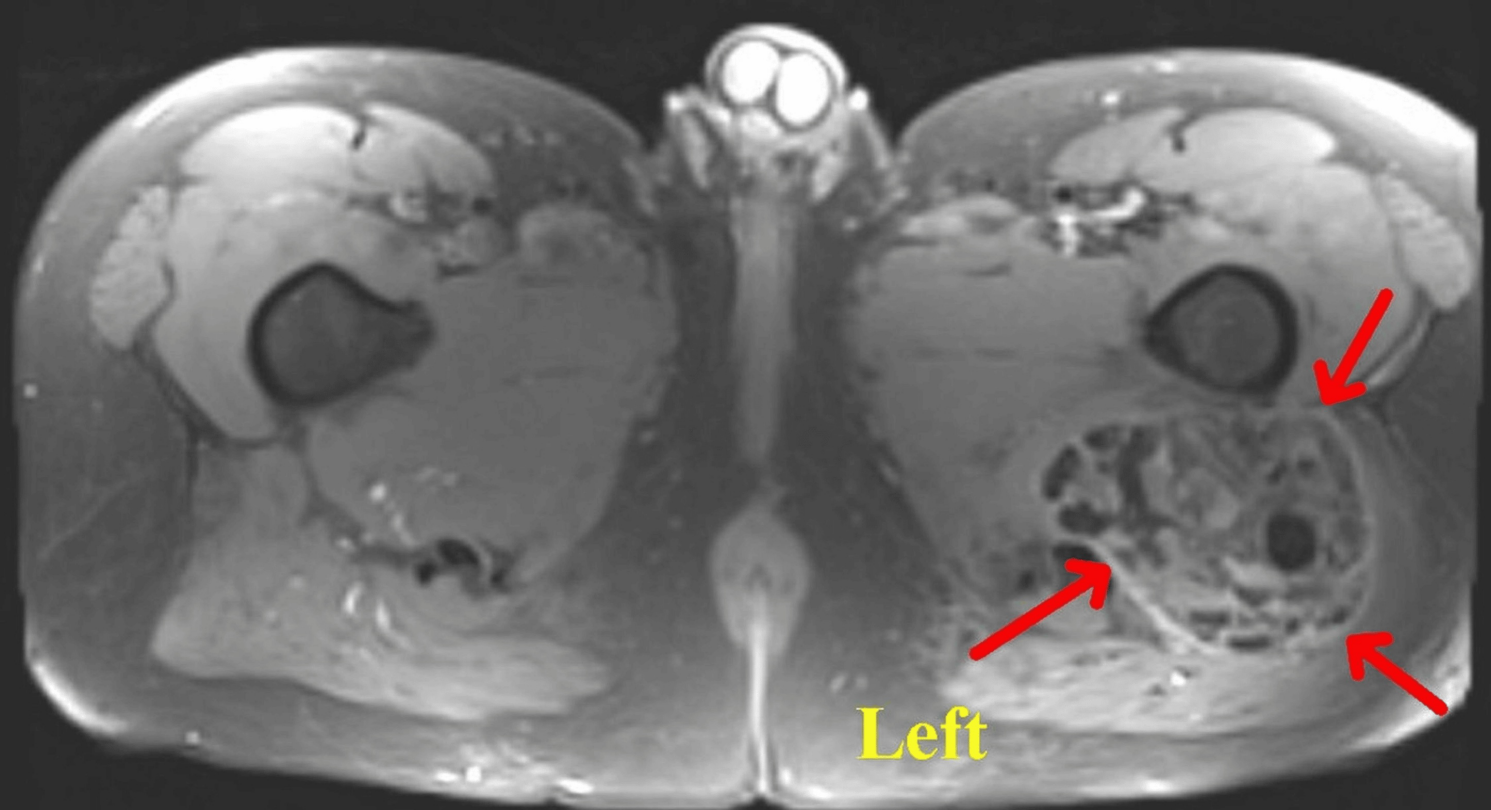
MRI hip cross section: Stable complex mass in the posterior left hip and ischio-femoral space with amorphous calcifications, likely tumoral calcinosis, and associated marrow edema in the proximal femur, possibly due to impingement from the mass The mass measures 15.7 x 9.0 x 9.2 cm. MRI, magnetic resonance imaging.
